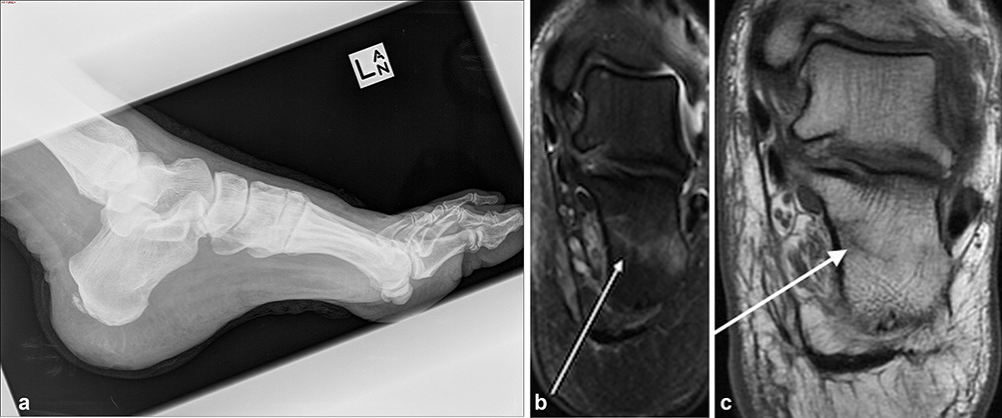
(a) Lateral radiograph of the left foot demonstrates a posterior calcaneal spur, but otherwise normal appearance of the calcaneum. (b,c) MRI coronal *T*_2_ fat suppressed sequence (b) demonstrates signal hyperintensity in the anterior calcaneus, which extends from the medial to the lateral cortex, with corresponding low signal on coronal *T*_1_ sequence (c). Findings are consistent with a non-displaced fracture. A soft tissue defect at the heel (not shown) does not extend to the calcaneal cortex. Remaining calcaneal signal (not shown) is normal with no evidence of osteomyelitis.
